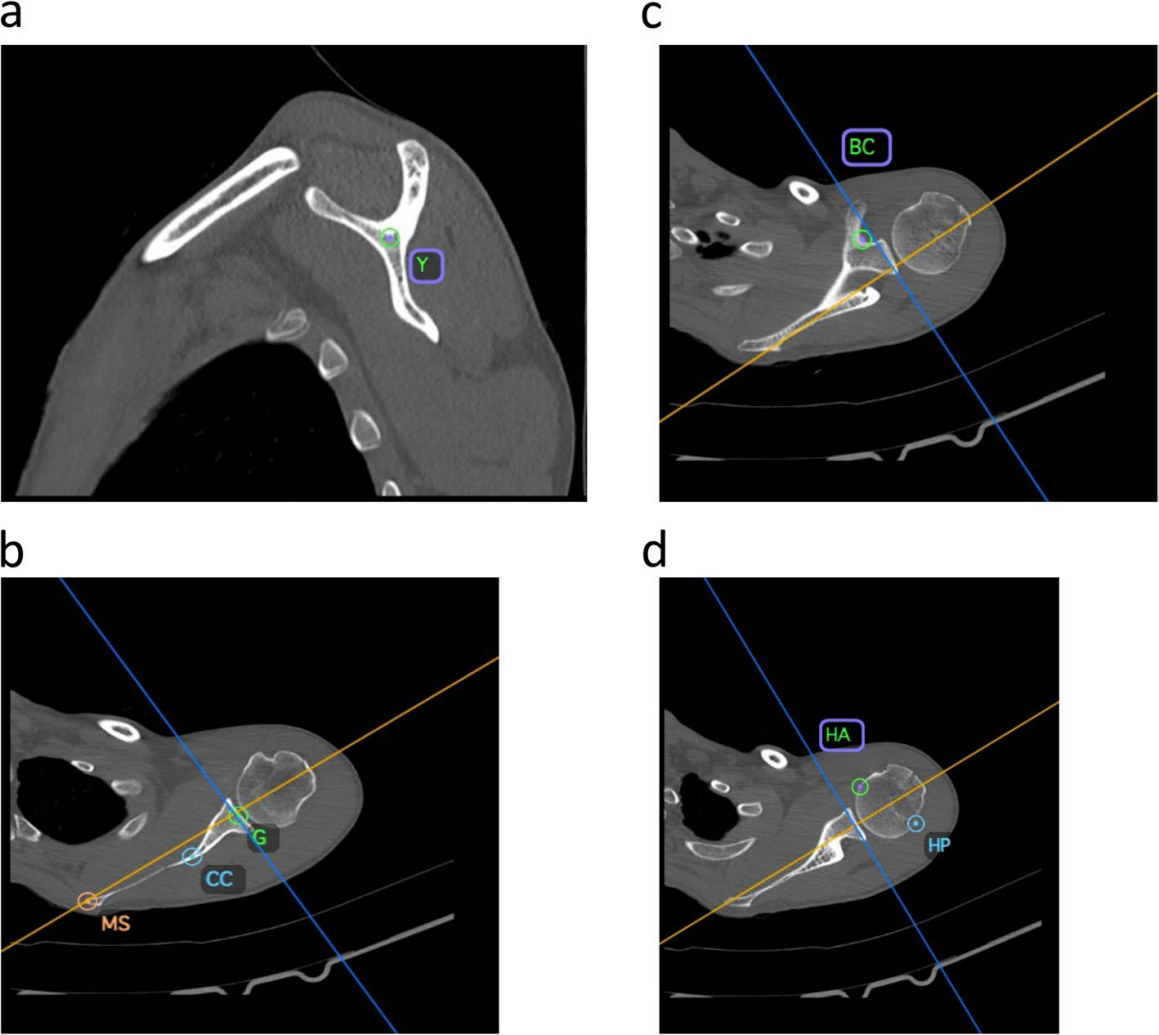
The 7 following points were digitized and named on each CT scan: a The Y point was digitized in the parasagittal view, at the intersection of the 3 bone branches (Y) on the aforementioned ‘Y-plane’; b The centre of the glenoid (G), the most medial point of the scapula (MS), and the cortical convergence (CC) of the anterior and posterior margins of the glenoid were all digitized in the same axial plane; c The base of the coracoid (BC) was digitized where the lateral cortex of the coracoid process merges with the cortex of the scapula in the axial view; d The anterior (HA) and posterior (HP) margins of the humeral head were digitized in the axial plane where the humeral head appeared to have the greatest size
